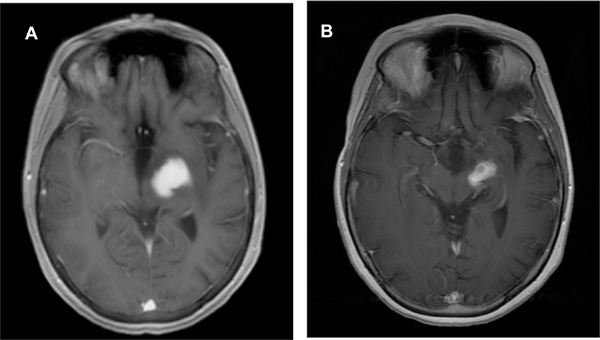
Ganglioglioma without identified MAPK pathway activation mutations demonstrates a significant and sustained response to MEK inhibition with trametinib T1-weighted post-contrast axial images: (A) showing contrast enhancing lesion centered in the left thalamic region. (B) Follow-up imaging 6 months post-initiation of trametinib demonstrated a significant partial radiographic response
